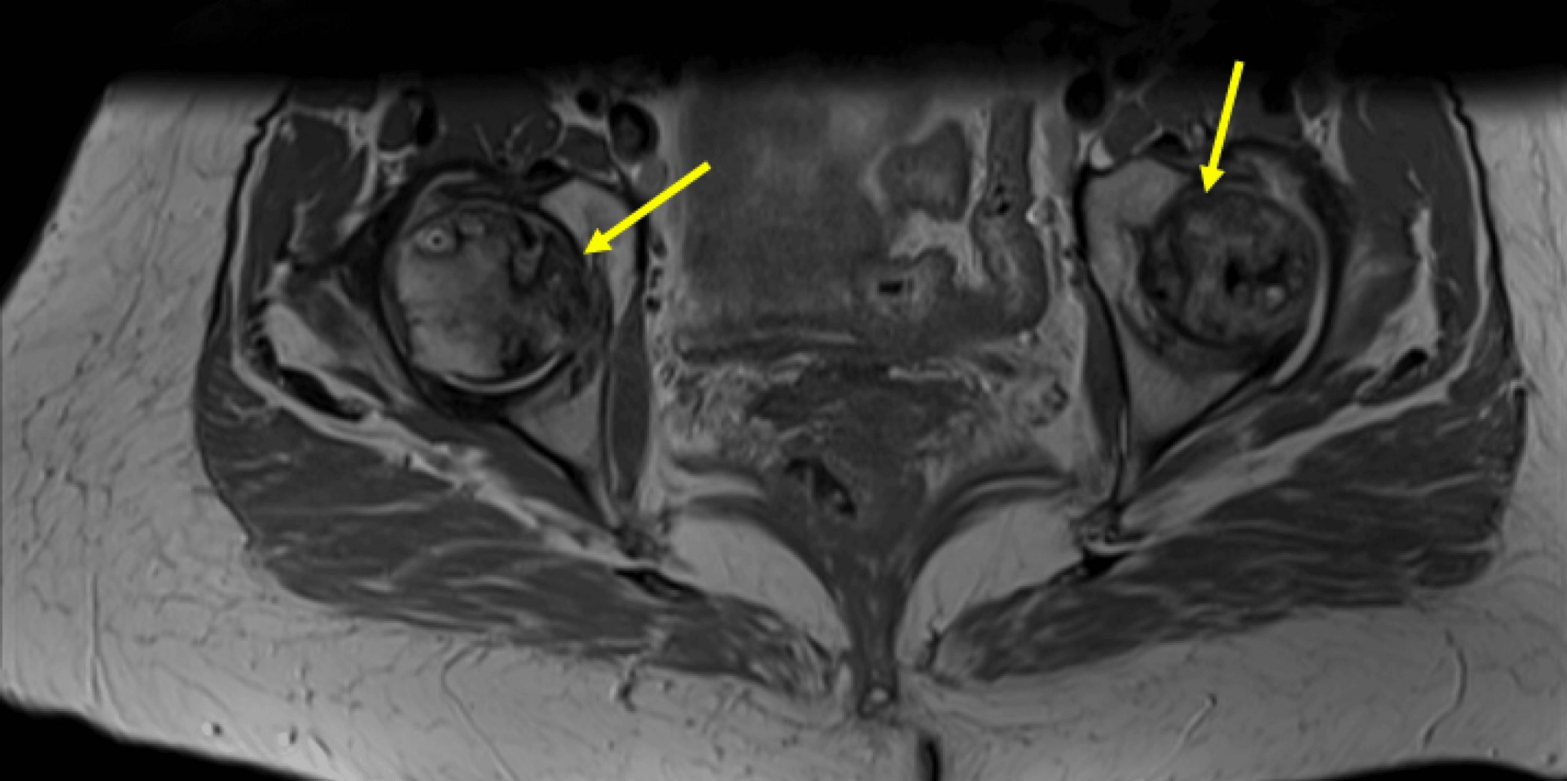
Bilateral femoral head osteonecrosis on MRI. Osteonecrosis of bilateral femoral heads in T1-weighted MRI images of the pelvis. Note the serpentine low-signal intensity bands (arrowheads) surrounding the central area of necrosis.
